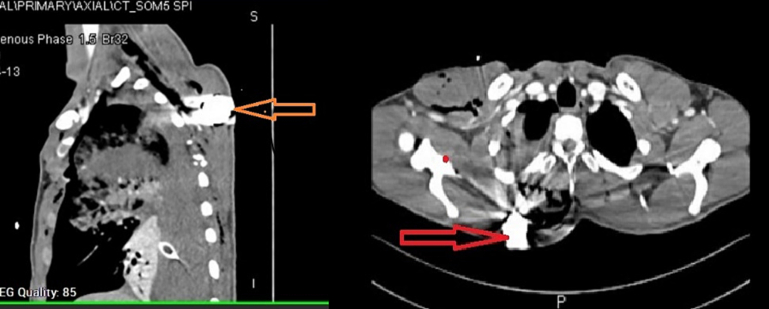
CT scan for case 3; arrows show the foreign body.
